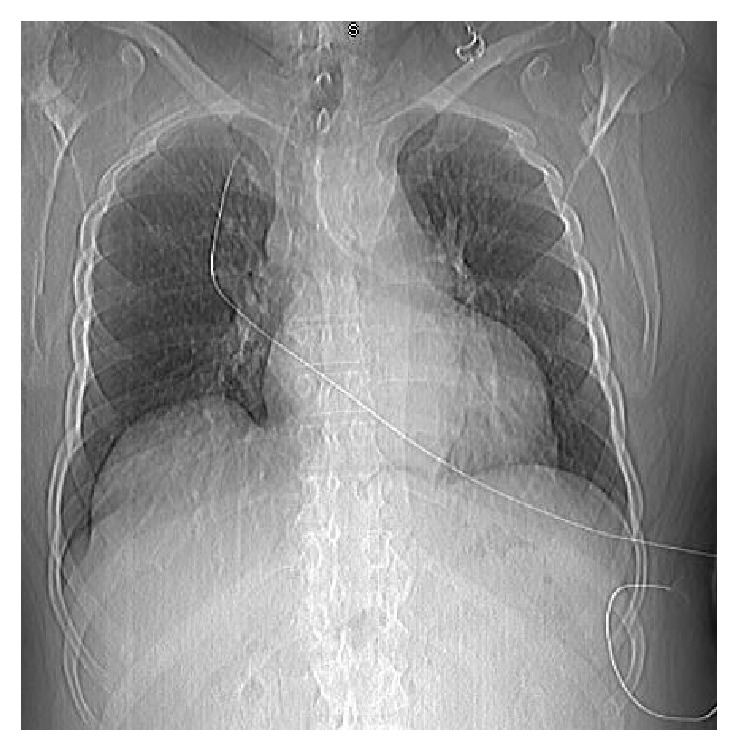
Chest X-ray showing prominence of thoracic aorta, no acute pulmonary disease.
